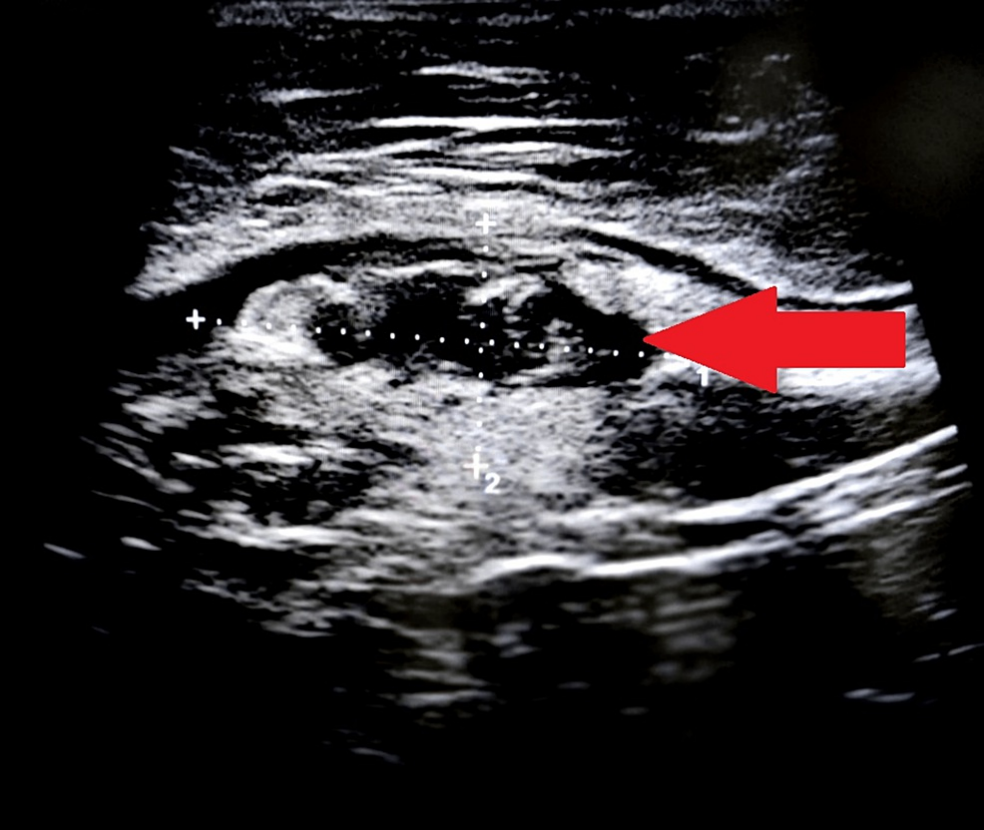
Ultrasound image showing peripheral compound cyst with a few septa.
